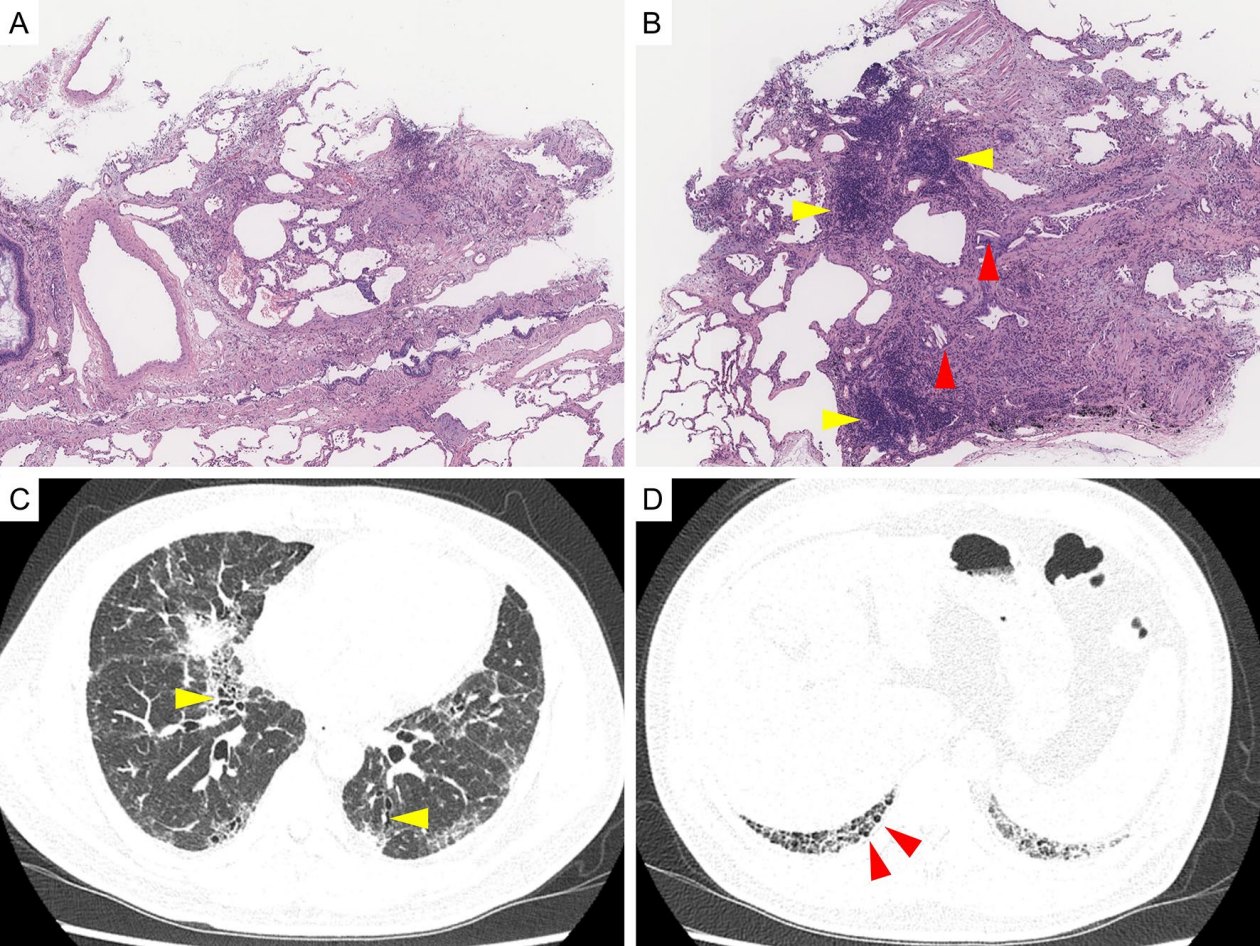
Another case in which the pathology diagnosis by TBLC was inconsistent with the diagnosis of MDD. (A), (B): pathology by TBLC. Dense fibrosis were observed. In addition, lymphoid follicles with germinalcenter (orange arrow) and giant cells with cholesterol clefts (red arrow) were observed. (C),(D): HRCT. Traction bronchiectasis (yellow arrow) and honeycombing (red arrow) were observed. But small centrilobular nodules or other findings suggestive of connective tissue disease associated interstitial lung diesase were not observed.
